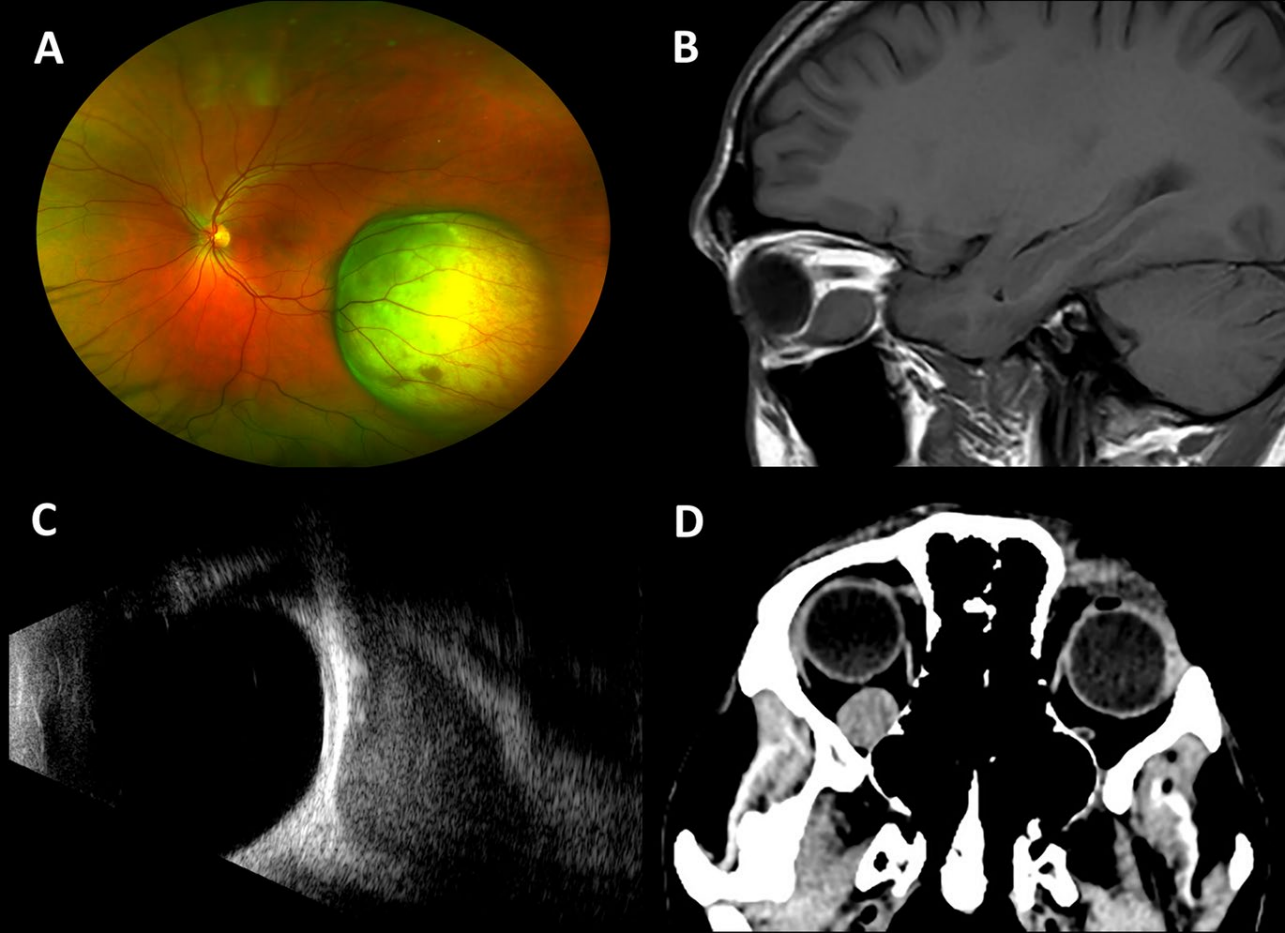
Multimodality imaging in a patient with orbital metastasis. A Fundus photography shows a choroidal melanoma in the left eye six years before the diagnosis of orbital metastasis. B MRI in the sagittal plane shows an enlargement of the inferior rectus muscle. C Ultrasound B-scan in longitudinal approach shows a low reflective enlargement of an extraocular muscle. D CT scan in the axial plane shows a well-outlined, right-sided orbital lesion, in the intraconal space
